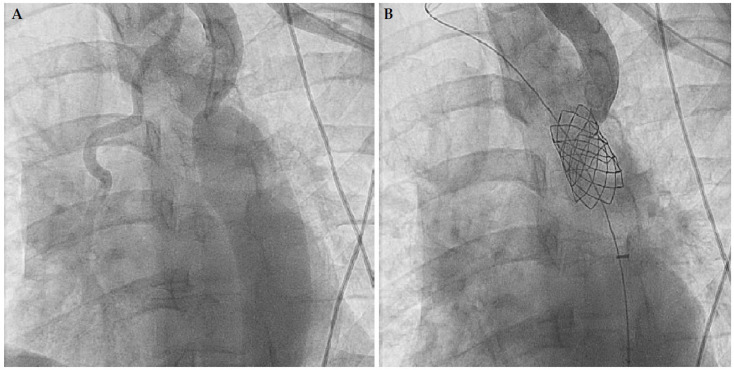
(A) Severe coarctation with collaterals and gradient of 30 mmHg across the coarctation segment in a 46-year-old adult male with hypertension, initial diagnosis on echocardiography (B) Treated with 45 mm CP stent with final stent diameter of 20 mm and complete resolution of coarctation gradient.
